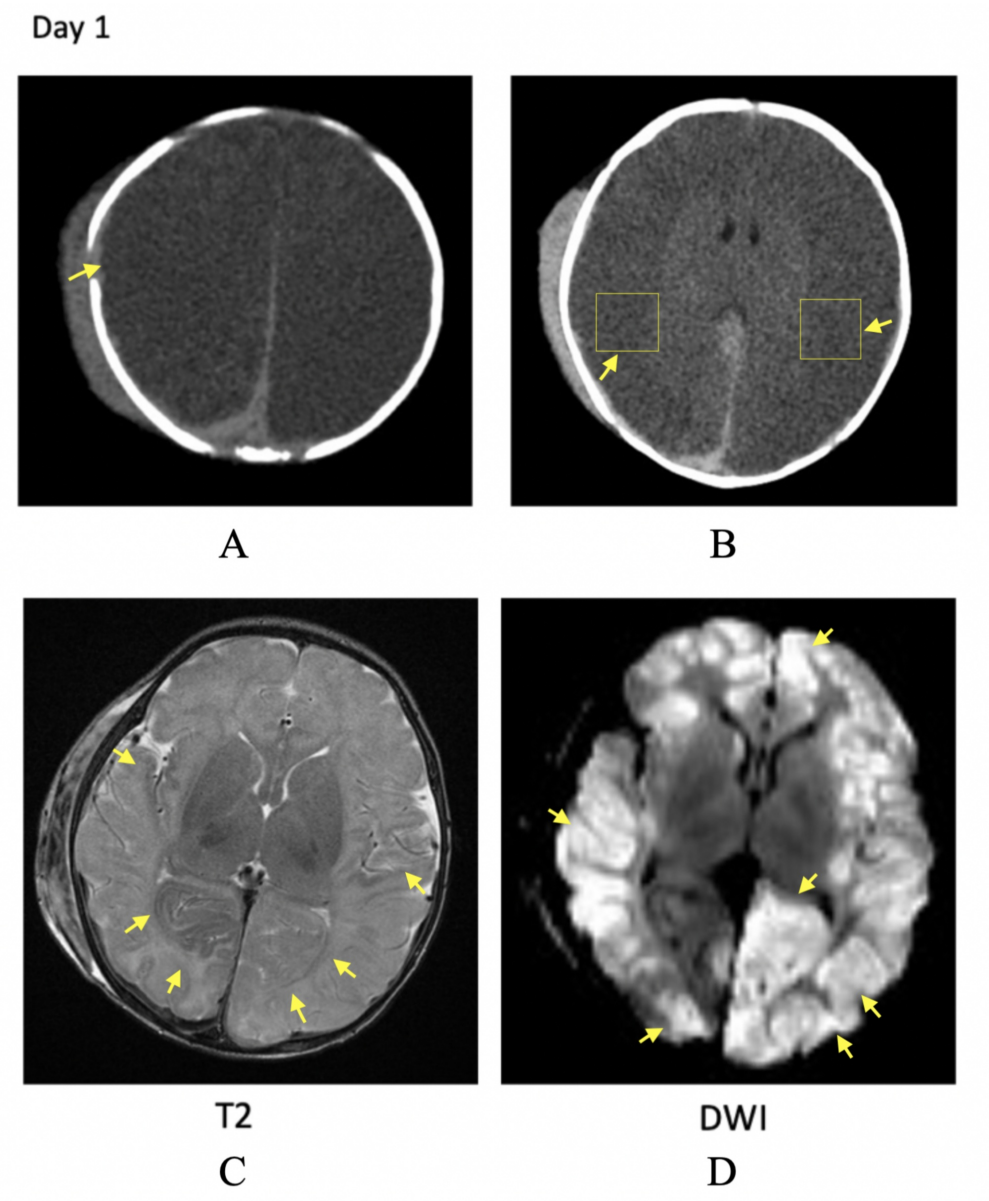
Radiological findings on day one of admission A: Head CT demonstrating displaced right parietal fracture; B: Head CT showing poor gray-white differentiation; C: MRI T2 showing diffuse pan-lobar injury; D: MRI DWI demonstrating extensive cortical diffusion restriction CT: computed tomography; MRI: magnetic resonance imaging; DWI: diffusion-weighted imaging
